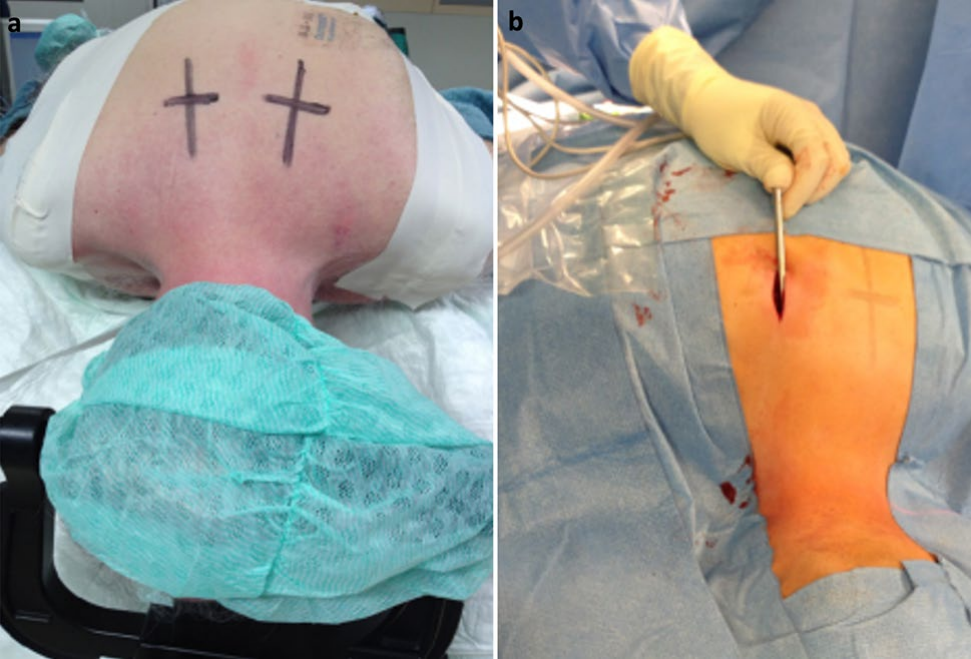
Intraoperative setting. After successful team time-out, a Mayfield clamp was applied, and the patient was positioned in a modified prone position, the so-called Concorde position. (a) First, the correct entry point for the skin incision is located with the aid of a K-wire and marked, usually at the level of TH 3/4 approximately 3 cm to the midline. (b) Subsequently, a 2 cm skin incision is made, and the fascia is opened. The drill guide is now advanced thrue the para spinal muscles and is placed on the lateral mass of C2. This is followed by fluoroscopically controlled drilling with constant monitoring for bone contact with the drill. When the drilling depth is sufficient according to the x-ray, the instrument provides the possibility of measuring the screw length. The screw can then be inserted through the guide instrument, again under fluoroscopic control.
